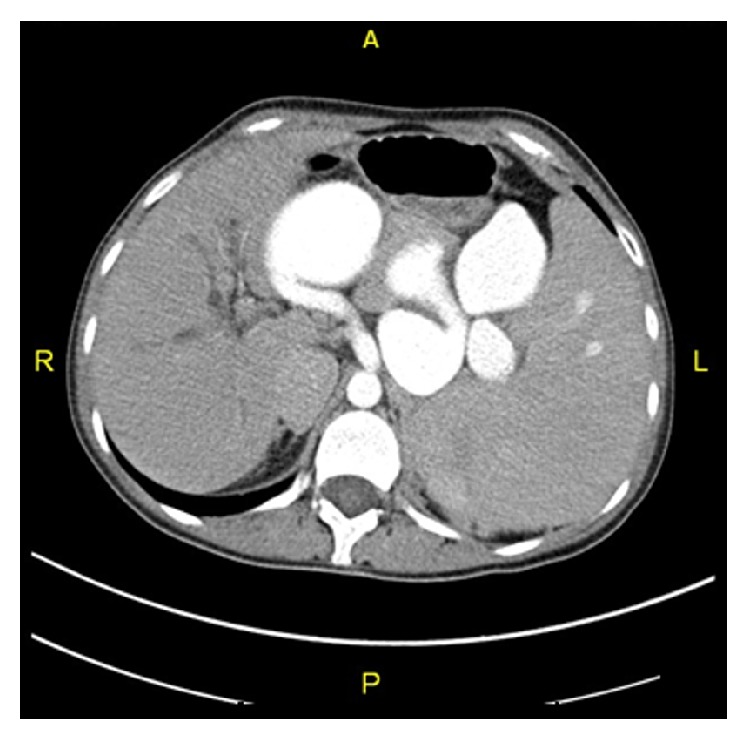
Computerized Tomography (CT) scan shows multiple aneurysms along splenic artery.
